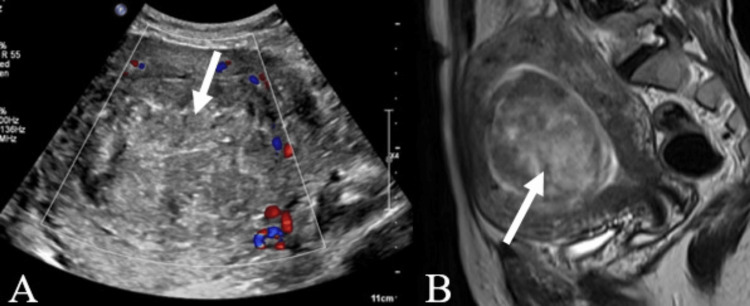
A case of a submucosal fibroid A: USG color Doppler image of the pelvis showing a heterogeneously hypoechoic lesion (white arrow) in the submucosal region of the uterus occupying the endometrial cavity showing peripheral vascularity. B: Sagittal T2-weighted pelvis MR image showing heterogeneously hyperintense lesion (white arrow) filling the endometrial cavity, consistent with submucosal leiomyoma. USG - ultrasonography
